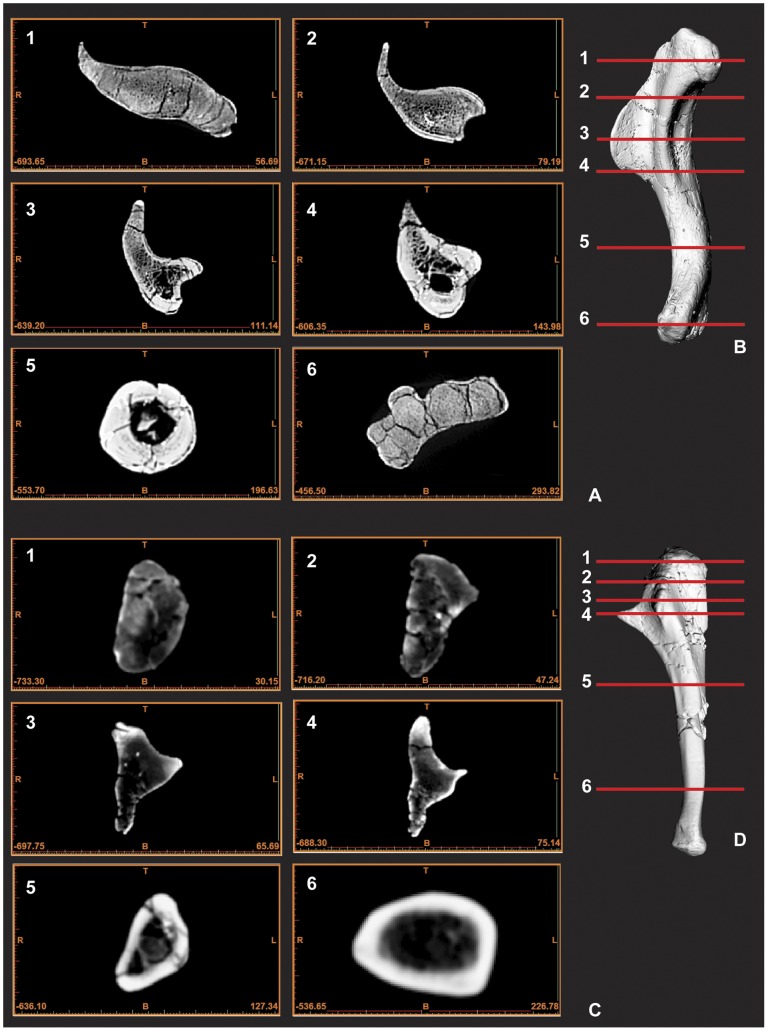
CT scan images of humerus and ulna displaying cross-section morphology. Humerus cross-sections images 1–6 (A), Computer generated render of humerus with corresponding image cross-section lines 1–6 (B), Ulna cross-sections images 1–6 (C), Computer generated render of ulna with corresponding image cross-section lines 1–6 (D). Scale is included as part of each CT image in mm.
